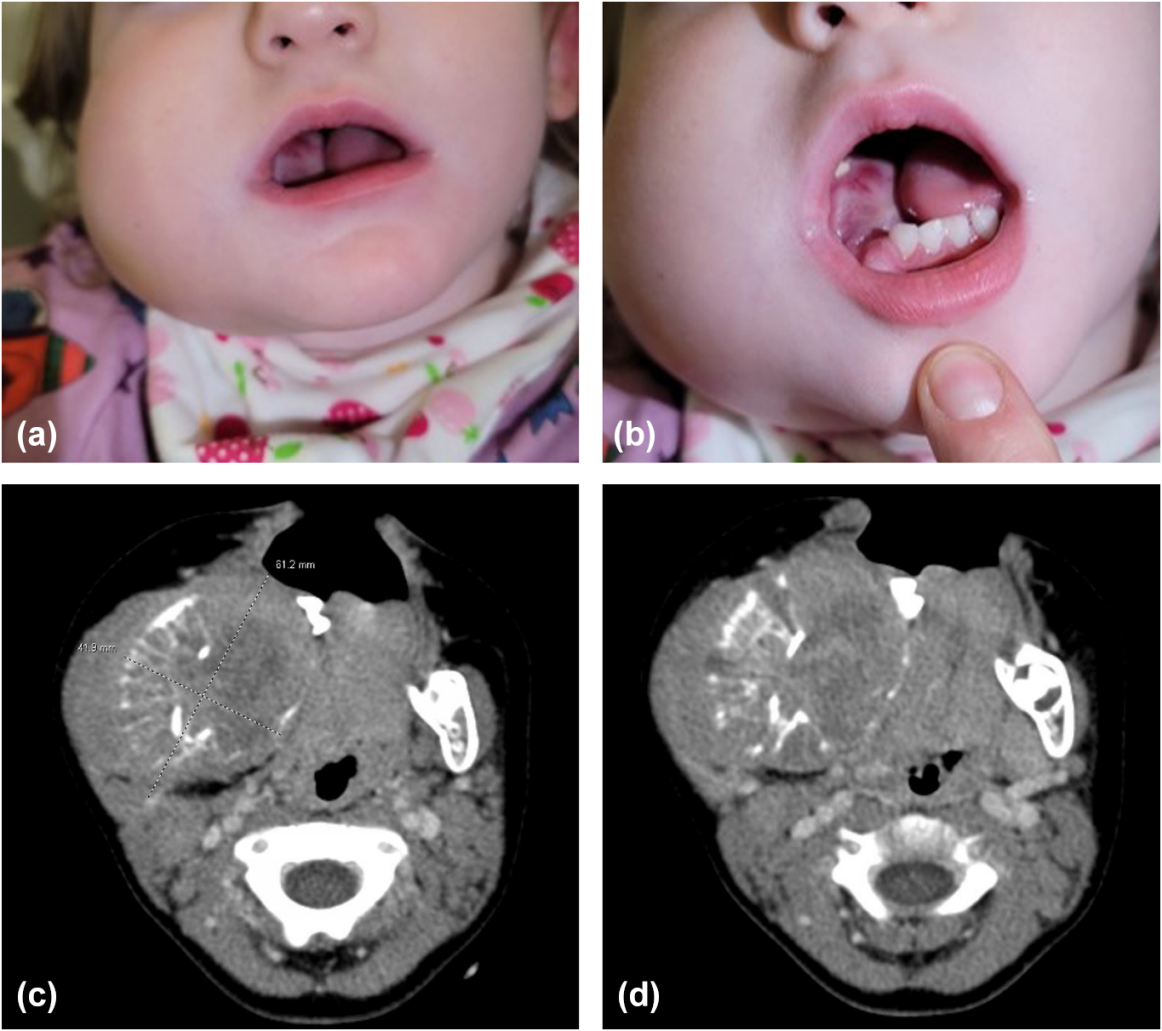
(a, b) Pre‐intubation photos of the patient's right mandibular mass, later diagnosed as metastatic neuroblastoma; (c) computed tomography (CT) scan of the patient's head and neck, where the right mandibular metastasis measures 6.1 cm at the widest diameter in the jaw; (d) CT showing midline shift but no local invasion of the patient's epiglottis.
